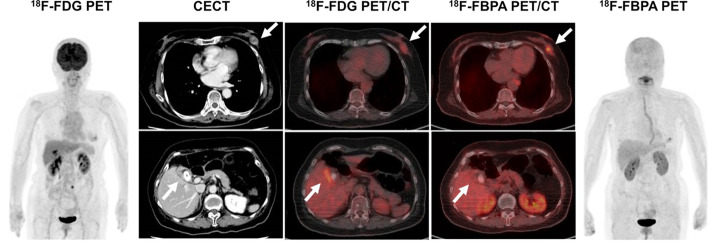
An 80-year-old woman underwent contrast-enhanced CT (CECT) for a recently diagnosed breast tumor before surgery. CECT revealed gallbladder wall thickening with gallstones and a contrast enhancement effect, in addition to a left mammary mass, which may indicate gallbladder cancer. ^18^F-FDG PET/CT showed a local increase in accumulation in the gallbladder wall (SUVmax: 8.1), making it difficult to differentiate gallbladder cancer from cholecystitis. ^18^F-FBPA PET/CT showed accumulation in the left breast mass (SUVmax: 4.5), but no significant accumulation in the gallbladder wall (SUVmax: 2.8). Cholecystectomy was performed and the pathological diagnosis was inflammatory granulation tissue
